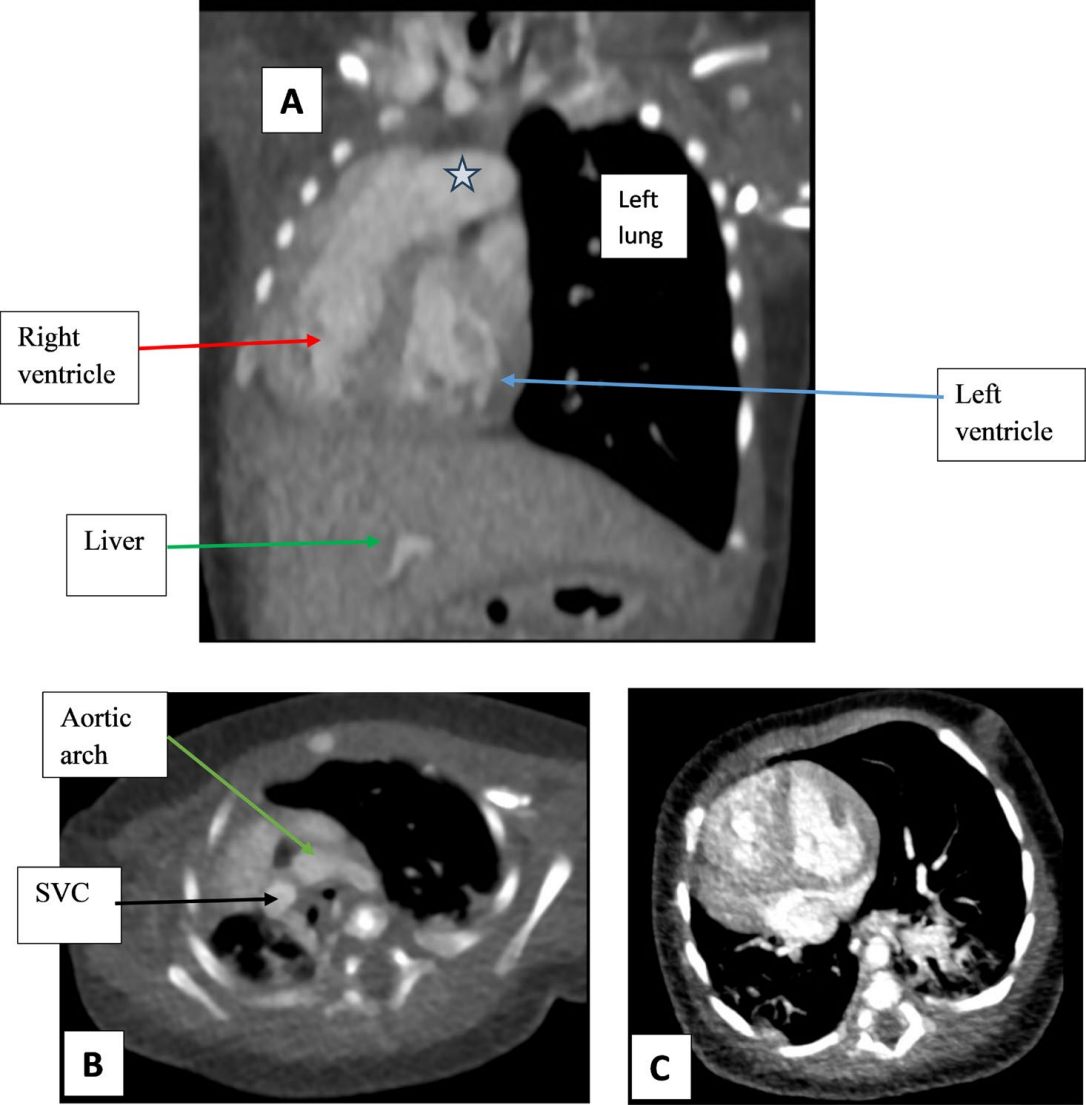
(A, B C) Post I.V contrast CT images of the chest, (A) Coronal reformat, (B, C) Axial cuts, soft tissue window. There is severe deficiency of the right lung with mediastinal shift to the ipsilateral side, a globally enlarged heart with a notably dilated main pulmonary artery (star in image A) but the levocardia is maintained with a left‐sided aortic arch noted and normal mono SVC. Image C shows the mediastinal shift and reduced right lung volume. There was no intracardiac shunt noted. No diaphragmatic defects or pleural effusions are noted.
